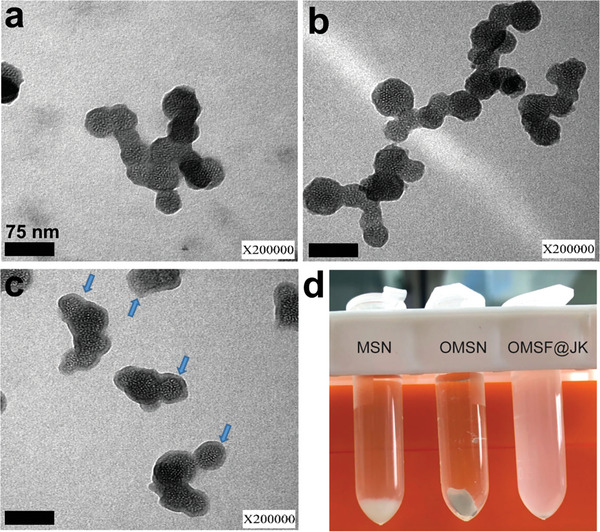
TEM images showing the morphology of a) MSN, b) OMSN, and c) OMSF@JK. MSN and OMSN showed an aspherical shape (30–50 nm in size) with a visible porous structure and maintained the surfaces‐porous in OMSN: and coated overall shape in OMSF@JK with a thin layer containing JK and PF‐127 (Scale bar: 75 nm). d) Digital photographs of particles suspended in PBS (pH 7.4). MSN, OMSN, and OMSF@JK were suspended in PBS at 1 mg mL^−1^ concentration ultrasonically (5 min, 80 w, water bath) and photographs were taken after 30 min of incubation at room temperature.
